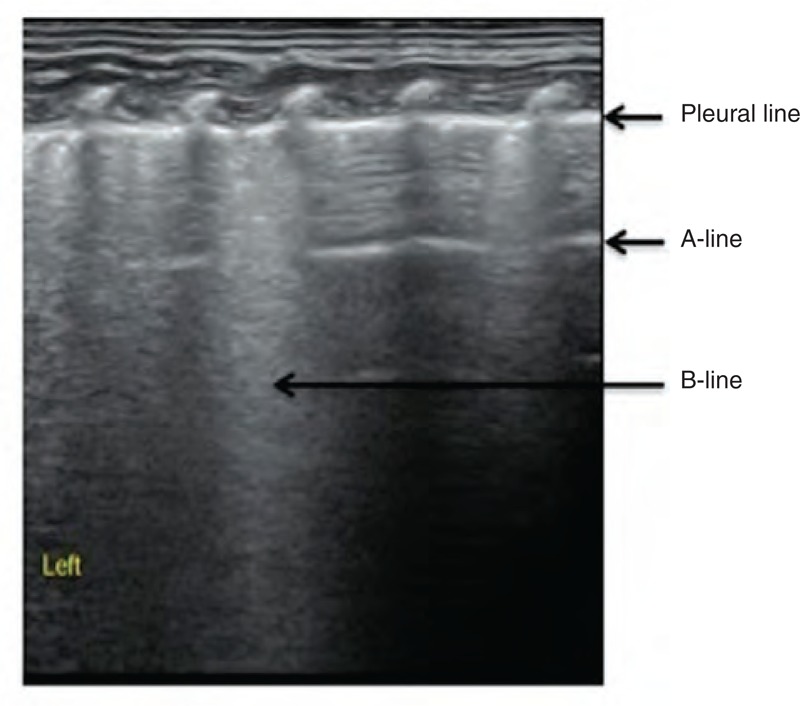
Interstitial syndrome. A lung ultrasound demonstrated interstitial syndrome in the upper field of the left lung, whereas the lower field of this lung was almost normal, suggesting the presence of mild lung edema.
